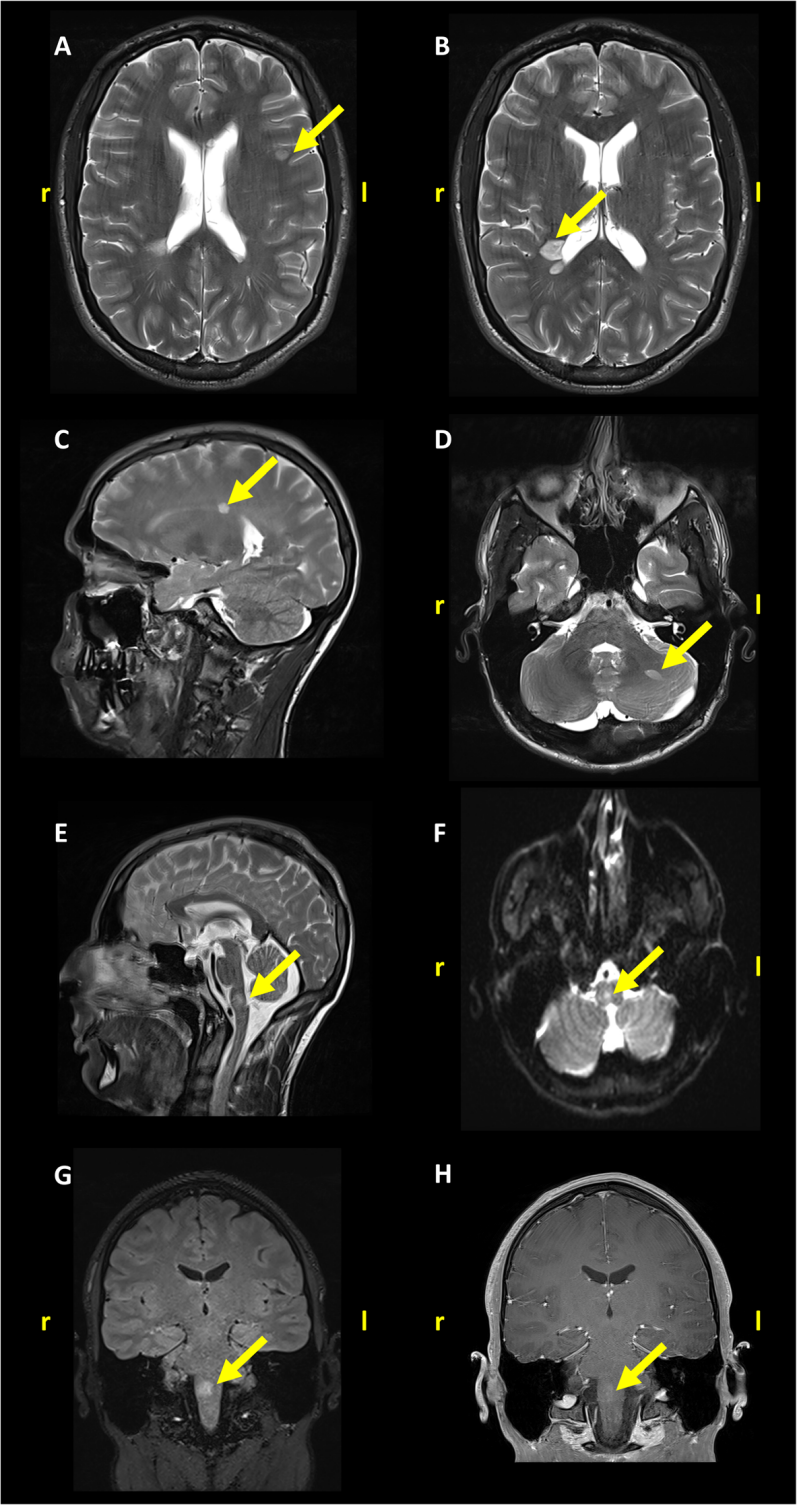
Brain-MRI shows multiple supratentorial (a-c) and infratentorial (d-h), as well as one spinal (not shown) lesions suggestive of multiple sclerosis. The lesion in the medulla oblongata (e-g) (12x10mm) shows Gd-enhancement and high signal on DWI. A,B,D transverse T2 TSE, C,E sagittal T2 TSE, F transverse DWI weight, G coronal T2-FLAIR, H coronal T1 VIBE
